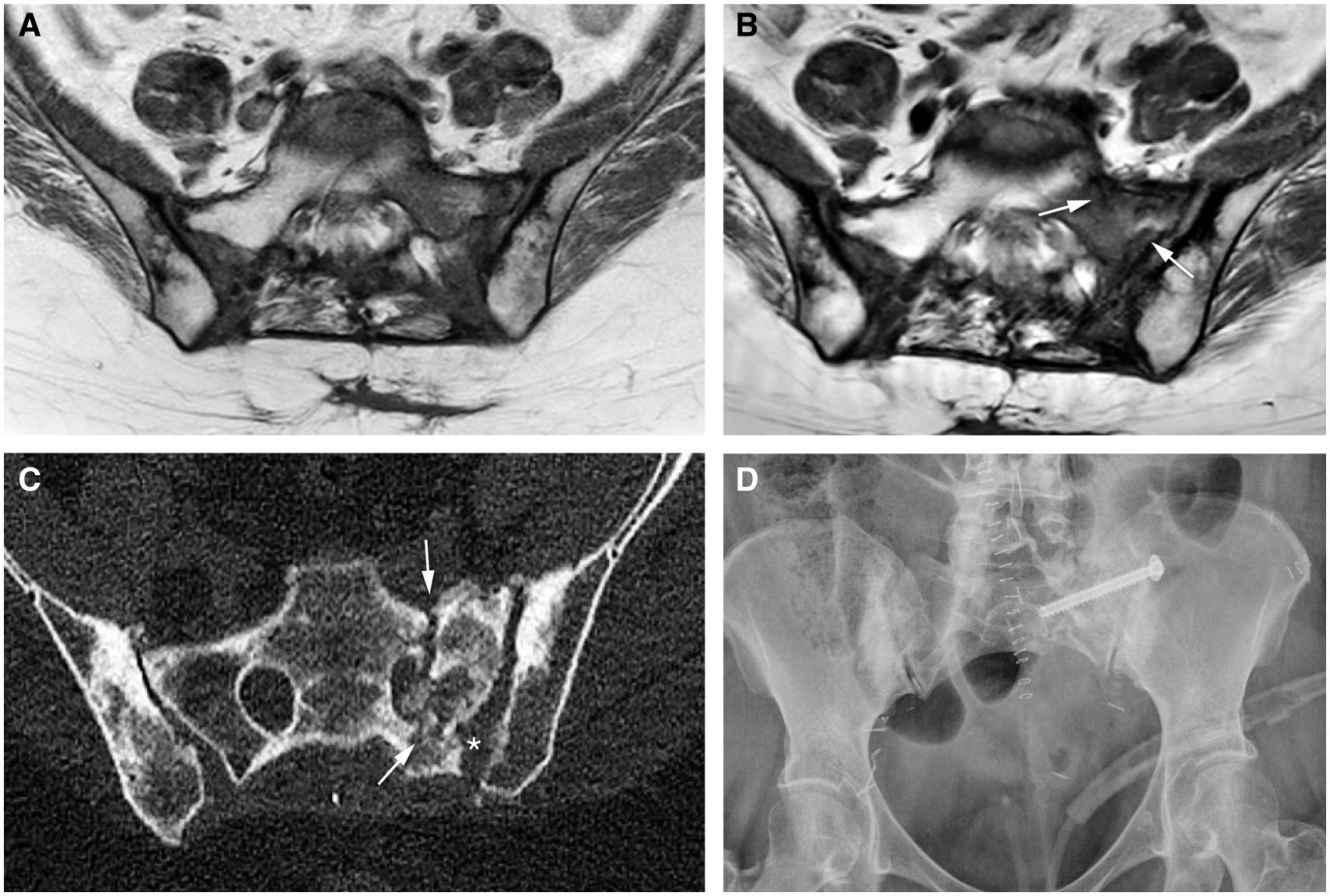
(A) Presence of BME-like changes but no clear fracture line on the left side of the sacrum is visible on the T1W MRI; (B) 18 months later, first appearance of the fracture line (*arrows*) on the T1W MRI; (C) CT scan prior to the stabilization surgery showing a complete fracture line in left part of the sacrum (*arrow*), a small fracture fragment (long *arrow*) is also seen with involvement of the neuroforamen S1 to the left. Diastasis in the left SI joint (*asterisk*) was also observed; (D) radiograph made 7 months following stabilization surgery showing the placement of a screw crossing the SI joint. BME = bone marrow oedema; CT= computed tomography; MRI = magnetic resonance imaging; SI = sacroiliac.
